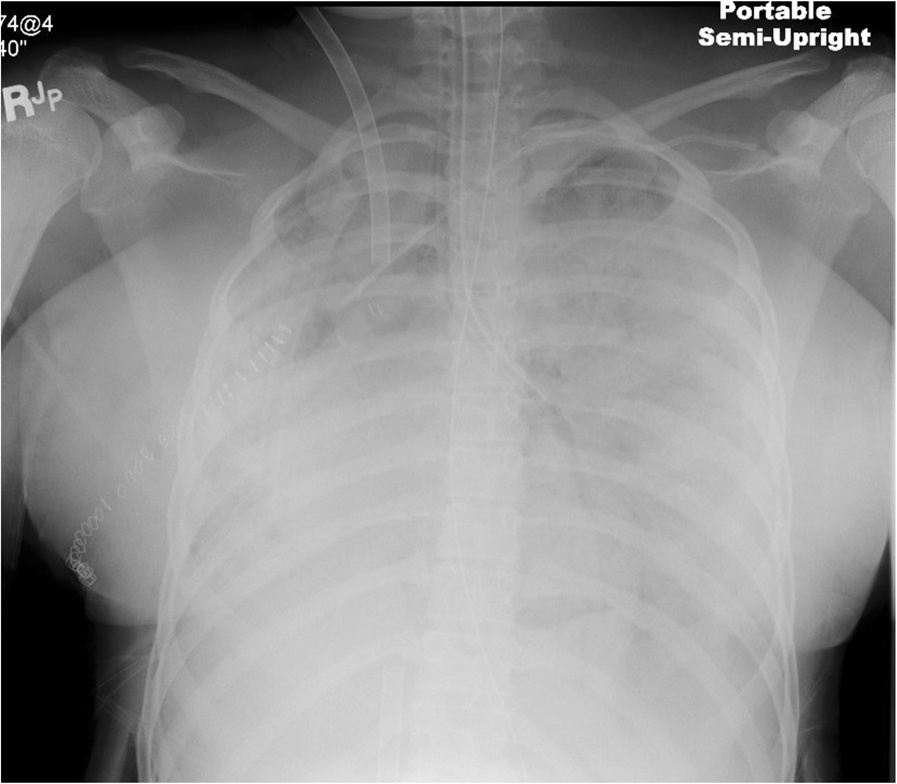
Patient chest x-ray. Patient lungs are shown to be clear.
